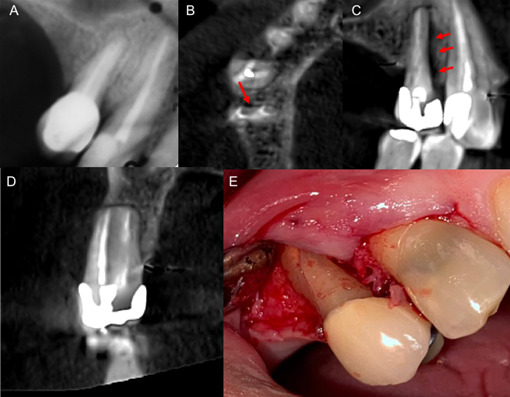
Caso 1. (A) Radiografía periapical donde se observan el ensanchamiento en el espesor del ligamento periodontal y moderado defecto óseo mesial. Cortes axial (B) y sagital (C) de tomografía computarizada de haz cónico que permiten evidenciar la amplitud del defecto óseo vertical mesial. Corte coronal (D) en el que se constata la ausencia de la tabla ósea vestibular. Fotografía clínica (E) obtenida durante la elevación del colgajo, que confirma la dehiscencia y la fractura.
